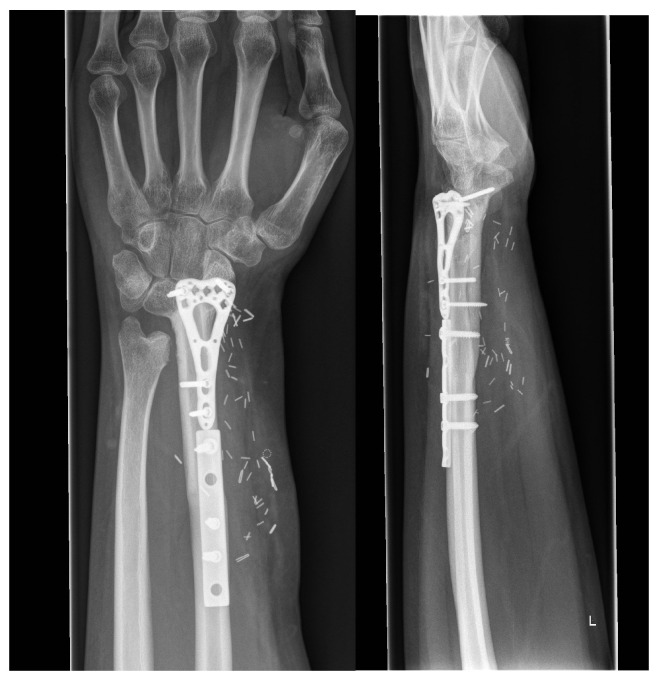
The X-ray taken 13 months later demonstrates the bony consolidation of the RFSL.
